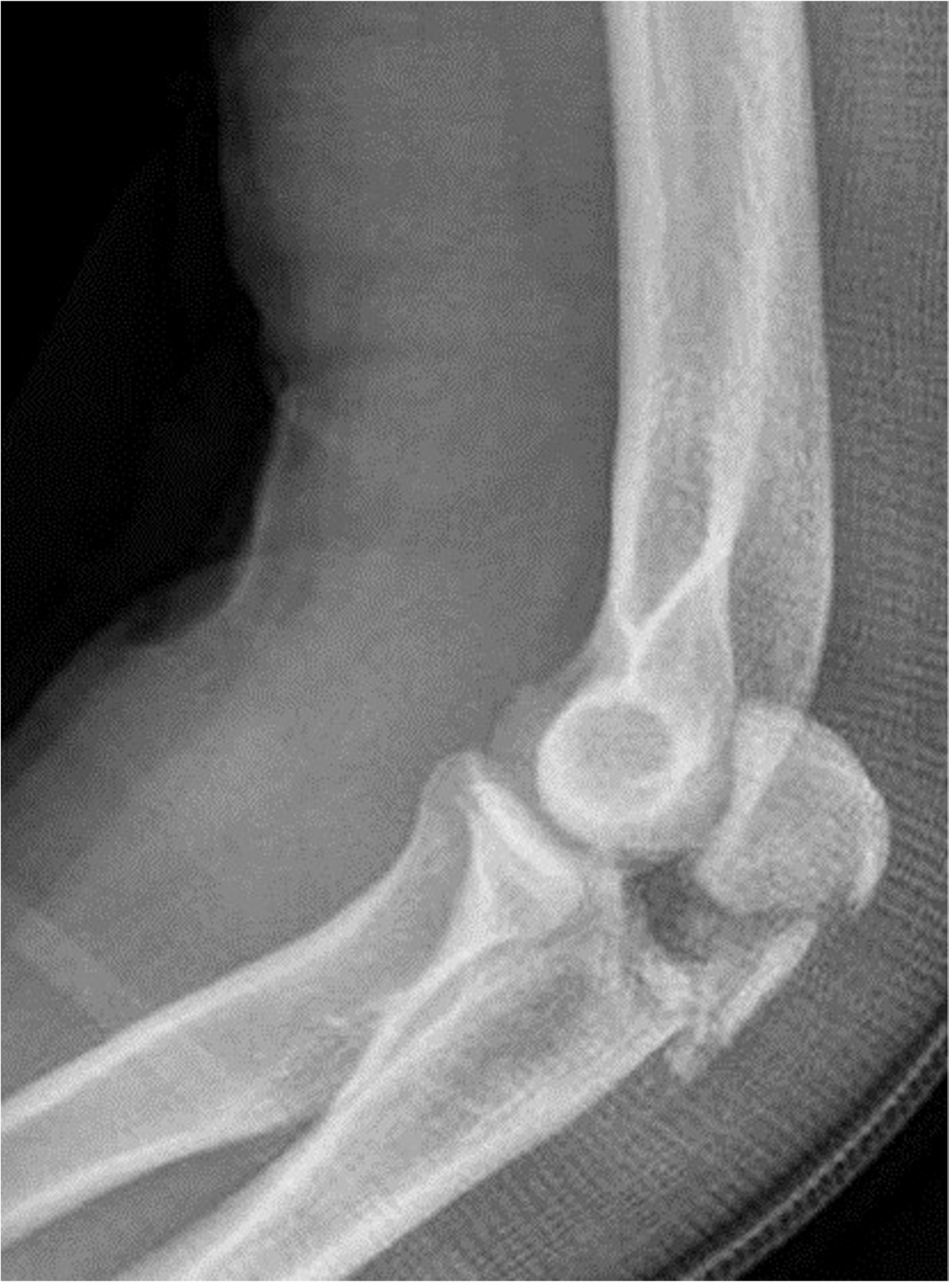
Preoperative lateral radiograph of an elbow with an olecranon fracture and comminuted articular surface
